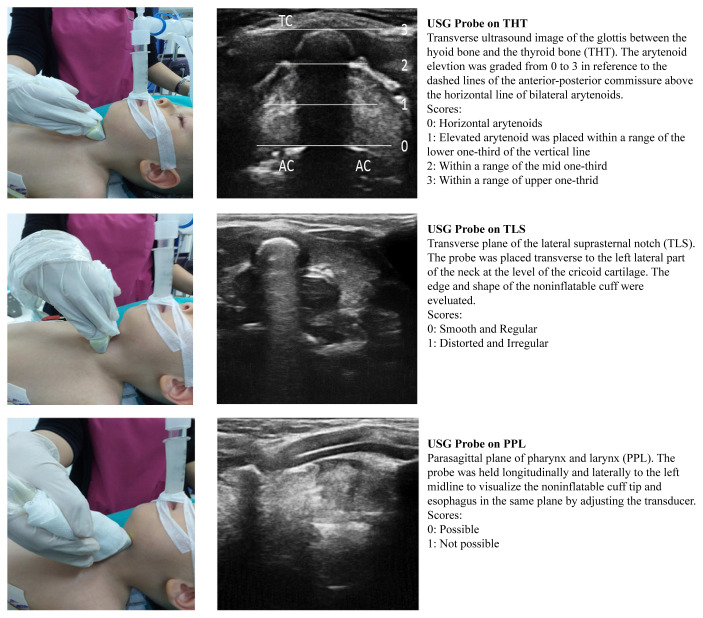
Figure showing the ultrasound airway examination and ultrasound examination scoring system in three different planes. AC = arytenoid cartilages, TC= thyroid cartilage.
